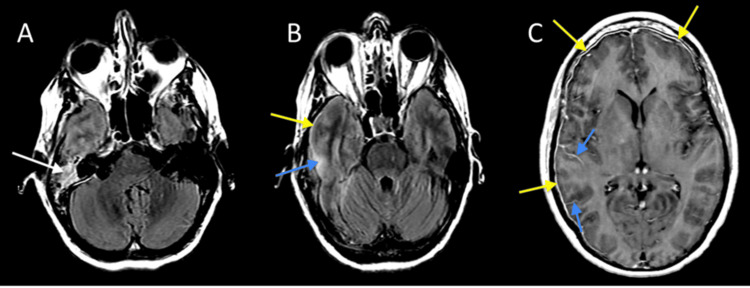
MRI brain with contrast (A) Axial T2 FLAIR MRI of the brain at the level of the temporal bones demonstrates right mastoid-middle ear hyperintensity (white arrow), consistent with otomastoiditis. (B) Axial T2 FLAIR MRI of the brain just superior to Figure 1A shows pachymeningeal thickening with hyperintensity (yellow arrow), consistent with pachymeningitis, sulcal, with adjacent temporal lobe parenchymal hyperintensity (blue arrow), indicating leptomeningitis and early cerebritis. (C) Axial contrast-enhanced T1-weighted MRI (Figures 1A-1B) demonstrates pachymeningeal (yellow arrows) and leptomeningeal (blue arrows) enhancement over the cerebral hemispheres, indicating pachymeningitis and leptomeningitis. FLAIR MRI, Fluid-Attenuated Inversion Recovery Magnetic Resonance Imaging
